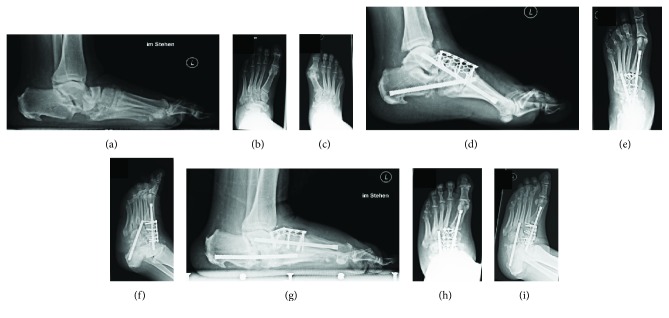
Radiologic case series of a 56-year-old male patient with IDDM for 19 years and severe midfoot Charcot arthropathy-type Sanders/Frykberg II/III on the left foot, with subluxation in the talocalcaneal and talonavicular joints. Initial diagnosis made in October 2013 after trivial distortion of the upper ankle joint (a–c) bearing radiographs in lateral, anterior-posterior, and oblique views. Intra- and extramedullary implants were used for reconstructive arthrodesis of the medial and lateral midfoot columns (d–f). Radiographs 6 months after surgery reveal a loss of reduction and implant breakage, displaying a frequent problem in Charcot surgery (g–i).
